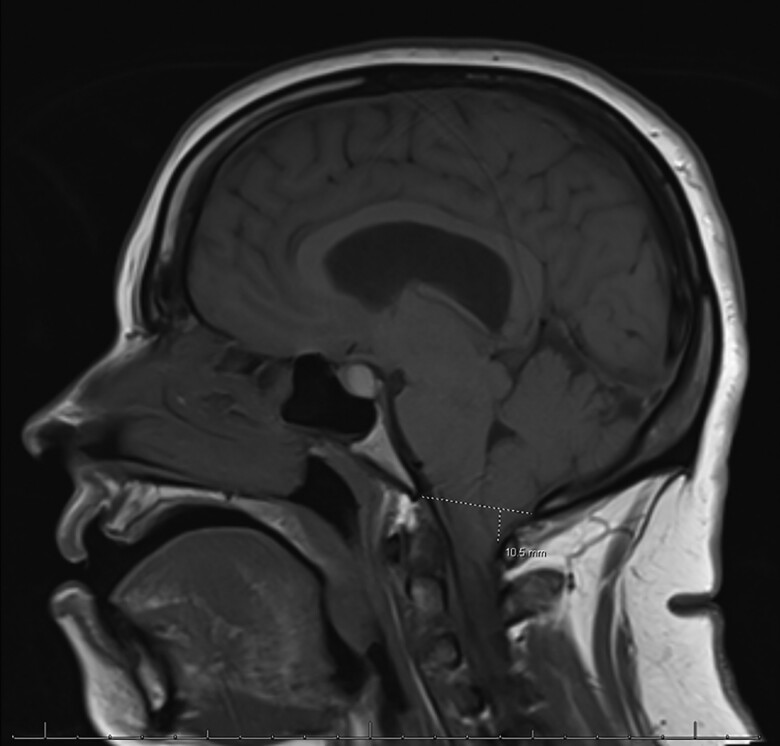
Magnetic resonance imaging brain with and without contrast; T1 sagittal image showing the cerebellar tonsils extending 10.5 mm inferior to the plane of foramen magnum compatible with Chiari I malformation.
